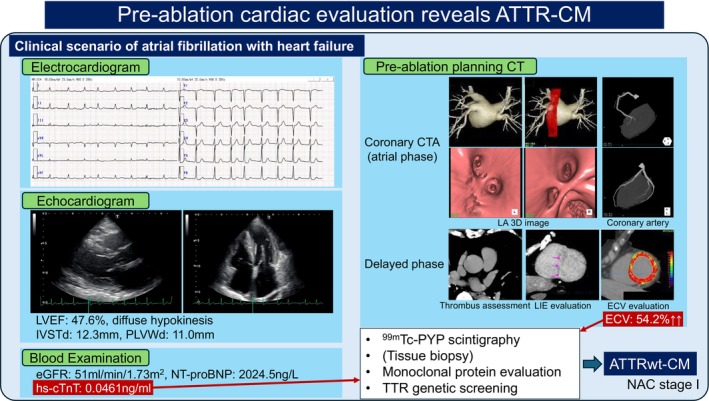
Pre‐ablation cardiac evaluation reveals ATTR‐CM. Clinical scenario of atrial fibrillation with heart failure is shown in this slide. ATTR‐CM, transthyretin amyloid cardiomyopathy; ATTRwt‐CM, wild‐type transthyretin amyloid cardiomyopathy; CT, computed tomography; CTA, CT angiography; ECV, extracellular volume; eGFR, estimated glomerular filtration rate; hs‐cTnT, high‐sensitivity cardiac troponin T; IVSTd, interventricular septal wall thickness at end‐diastole; LA, left atrium; LIE, late iodine enhancement; LVEF, left ventricular ejection fraction; NT‐proBNP, *N*‐terminal prohormone of brain natriuretic peptide; PLVWd, posterior left ventricular wall thickness at end‐diastole; TTR, transthyretin; 3D, three‐dimensional; ^99m^Tc‐PYP, technetium 99 m pyrophosphate.
